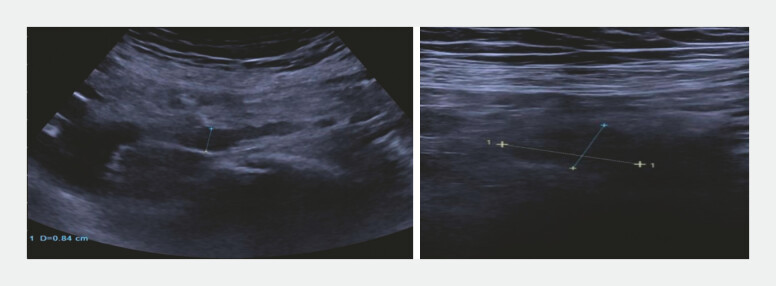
The abdominal ultrasound showed a strip hypoechoic area in the right lower abdomen and enlarged para-appendiceal lymph nodes.
